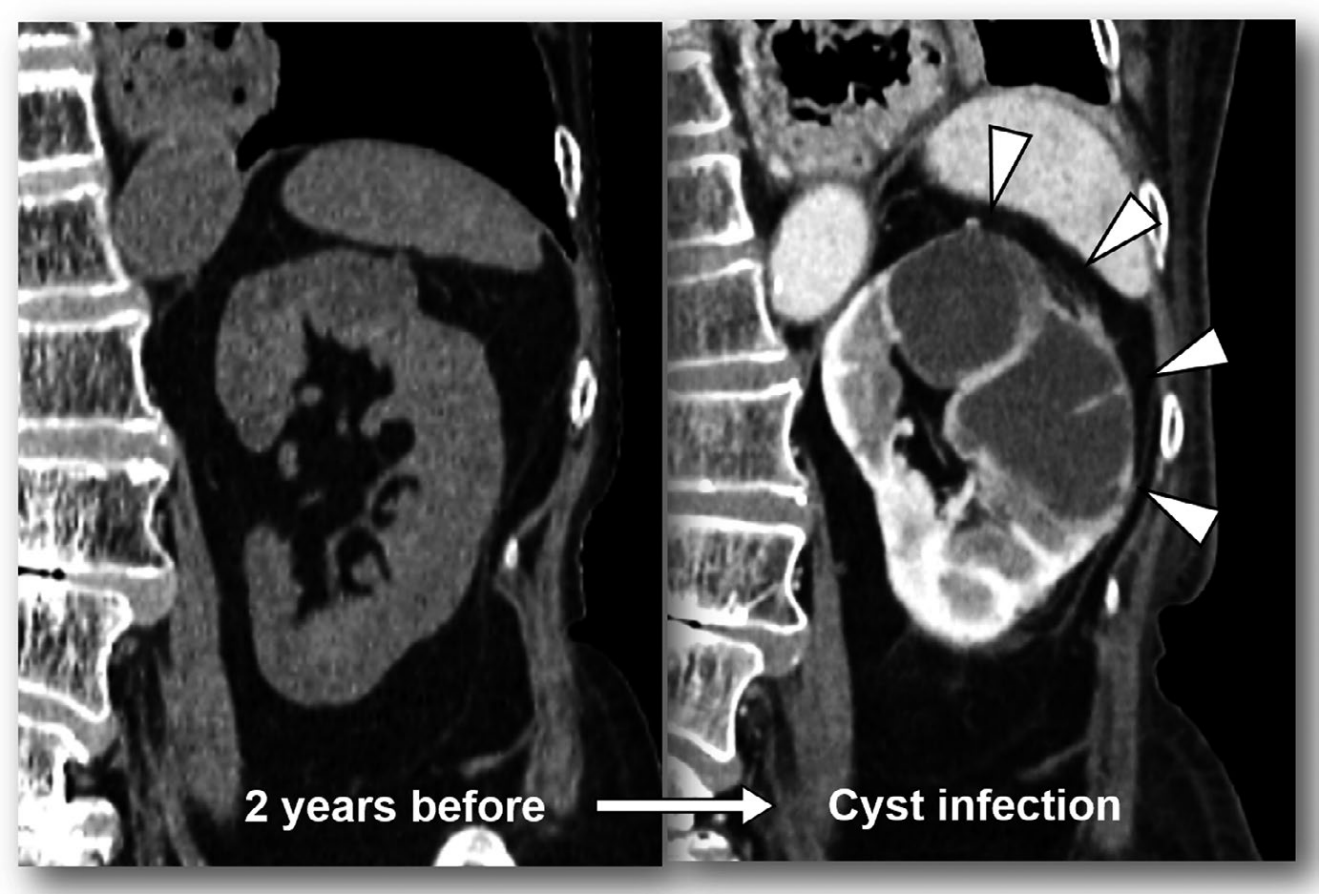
Compared to two years before, enhanced CT revealed that left renal cysts were enlarged with perinephric panniculitis (arrowheads), indicating infectious renal cysts
